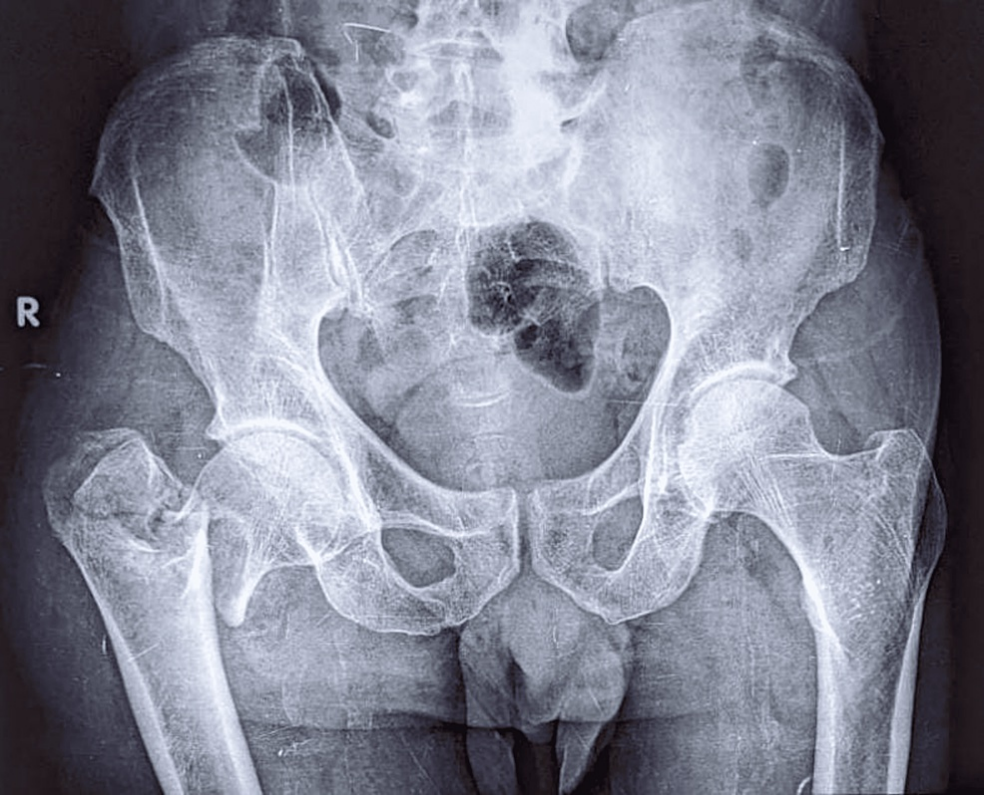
Preoperative radiograph showing unstable intertrochanteric fracture of the right femur
